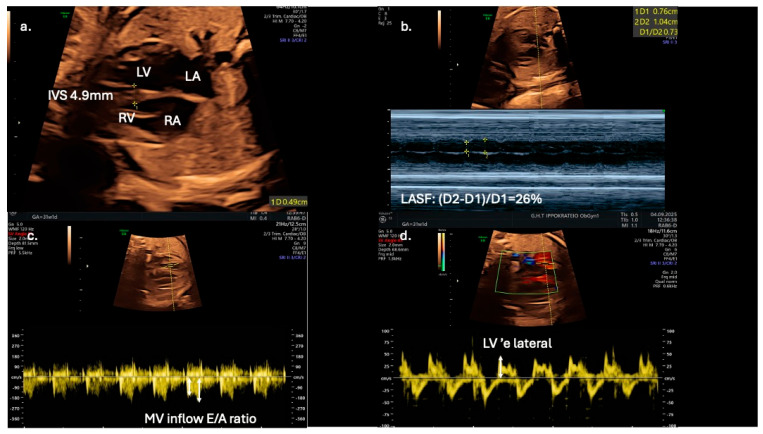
Echocardiographic indices in a 31-gestational-week fetus of a diabetic mother; (a) interventricular septal thickness (IVS) measured in the four-chamber view; (b) in the same view, left atrial systolic shortening (LASF) is calculated using M-mode echocardiography; (c) use of pulse wave Doppler imaging in mitral inflow to calculate E/A ratio; (d) application of tissue Doppler imaging in the lateral wall of the left ventricle enables further assessment of diastolic function by calculating ‘e wave and E/’e ratio.
